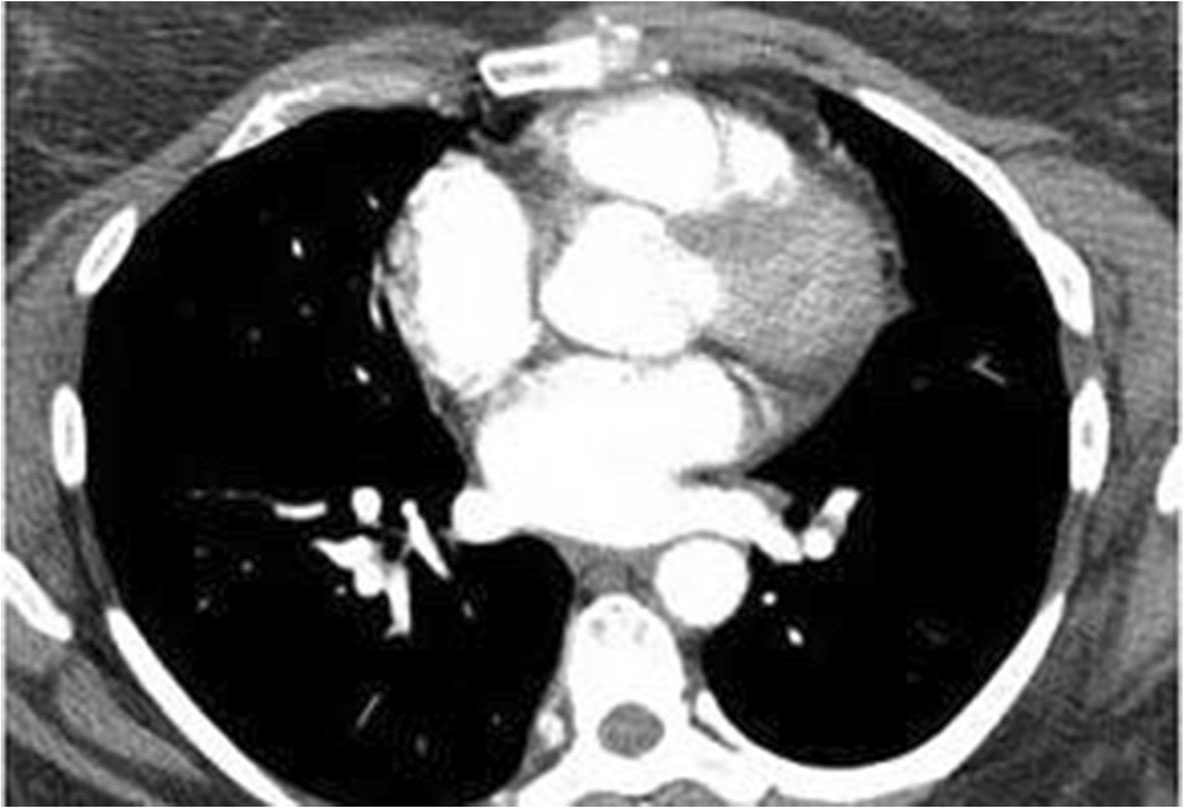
Computed tomography angiography of the chest without any evidence of emboli. A follow-up computed tomography after discontinuation of novel oral anticoagulant therapy and initiation of closely monitored conventional warfarin anticoagulation (international normalized ratio 2.0–3.0). A coronal section of this study at the same level as Fig. 1 reveals vessels filled with intravenous contrast indicating patency without the presence of emboli
